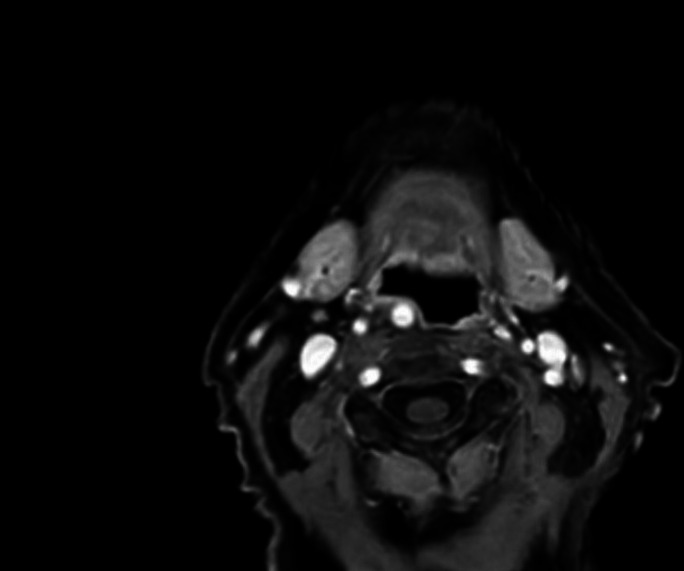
MRI, axial view. Impression of the right-sided ICA displacing the hypopharynx
